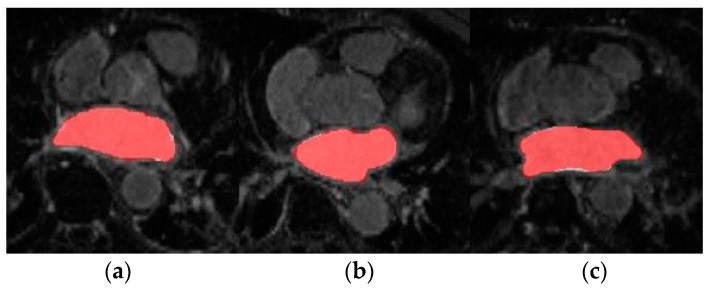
Axial slices of LA segmentations for three representative patients (a–c) obtained through the LGE-MRI source model. Ground-truth mask in white, prediction mask in red and intersection in pink.
